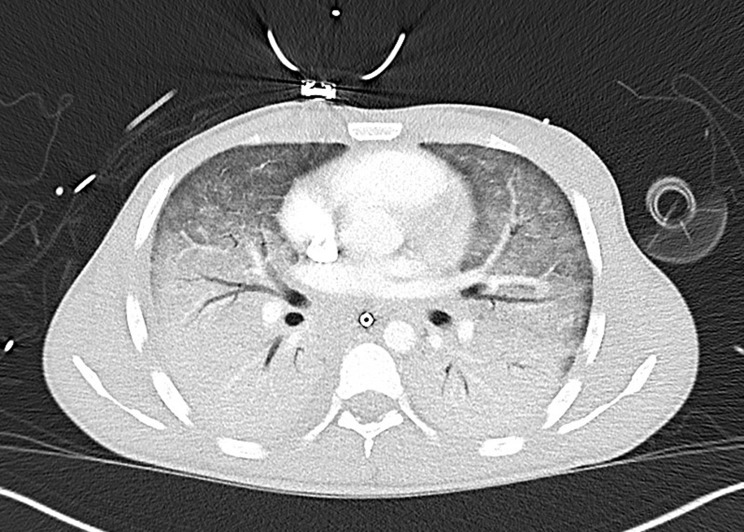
Chest CT at transfer to the university hospital. Progressive ARDS with increasing bilateral consolidations and ground-glass opacities, predominantly in the lower lobes. Likely reactive lymphadenopathy is present
